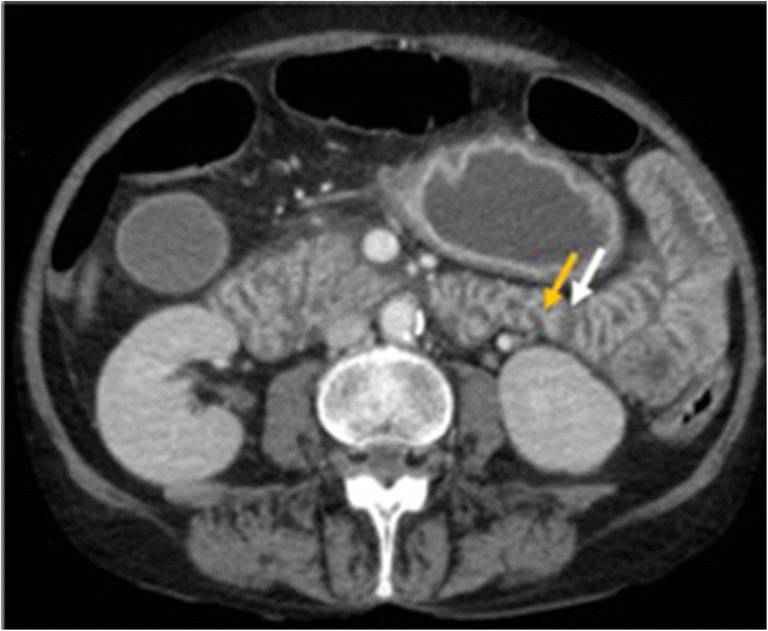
Shock bowel. Contrast-enhanced axial CT images in 56-year-old male with severe head trauma and bleeding from a large scalp laceration. Findings of shock bowel include diffuse intense mucosal enhancement (orange arrow) and submucosal oedema (white arrow)
